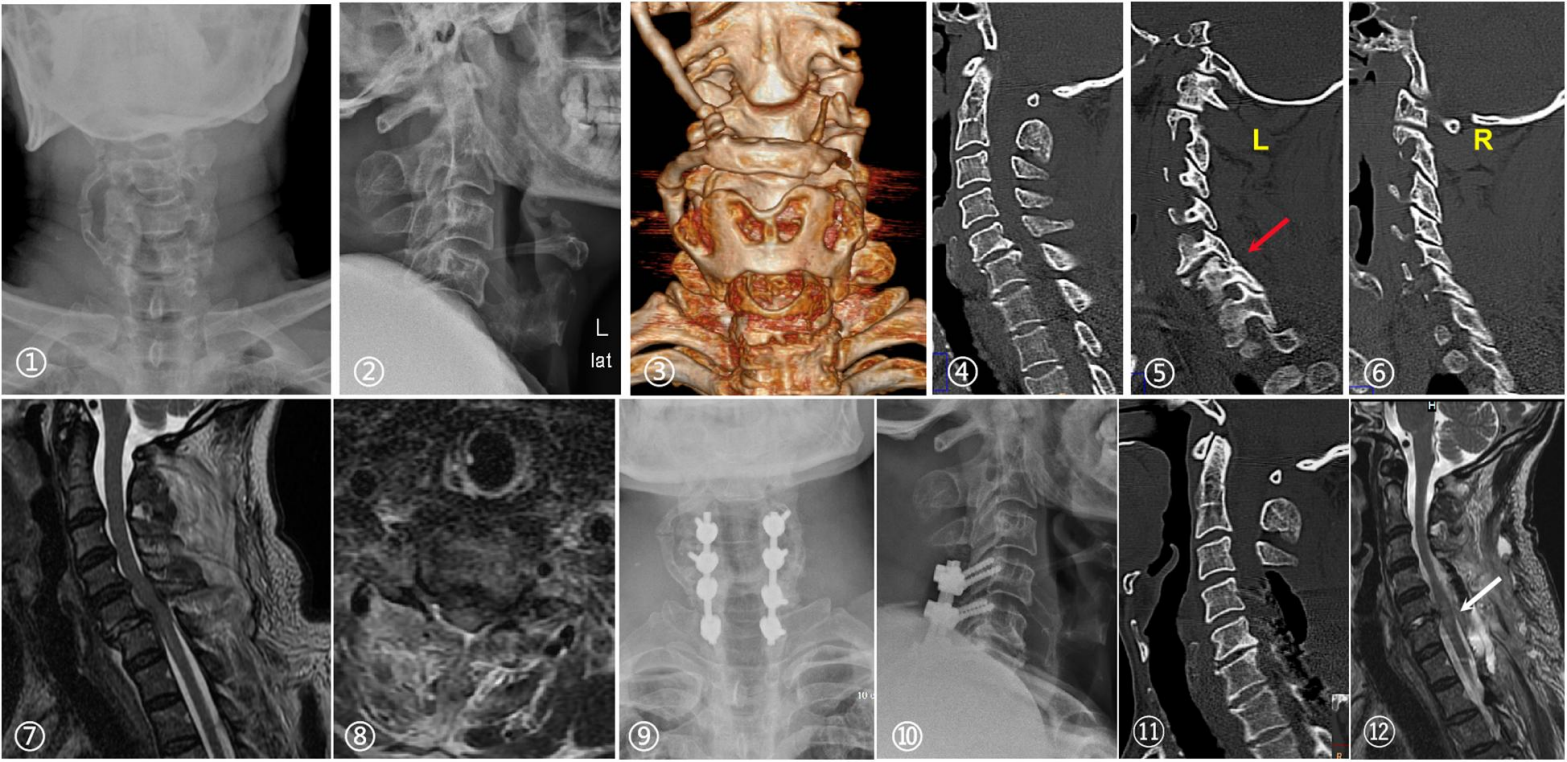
A 69-year-old male presented in July 2018 with “neck pain and restricted movement following a fall 12 h prior.” imaging confirmed a C5/6 fracture-dislocation with spinal cord injury (ASIA-D) and left facet dislocation with fracture [(2), (4), (5), (7), (8)]. An anterior approach was initially planned; however, bony fusion between the thyroid cartilage and hyoid bone [(1), (3)] prevented safe anterior retraction of the trachea and esophagus, necessitating a posterior approach. Preoperatively, the patient had largely recovered neurologically, so only facet joint unlocking, reduction, and posterior fixation were performed—without laminectomy or decompression. Following anesthesia recovery, the patient developed quadriplegia and decreased superficial sensation (ASIA-B). Emergency reoperation was performed with posterior laminectomy and extended decompression. Postoperative cervical x-rays [(9), (10)] and CT [(11)] confirmed C4–C6 laminectomy. MRI demonstrated signal changes in the spinal cord at C5/6 and confirmed adequate decompression [(12)].
